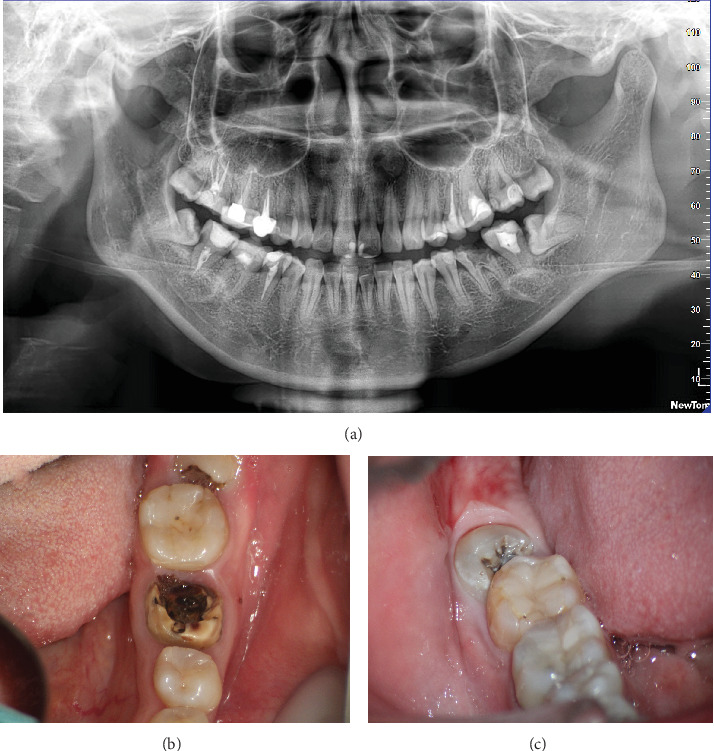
Preoperative panoramic radiograph and photographs. (a) Panoramic radiograph shows an unrestorable Tooth #19. The root of Tooth #32 is fused, making minimally invasive extraction available. (b) The preoperative clinical picture of Tooth #19. (c) The preoperative clinical picture of Tooth #32.
